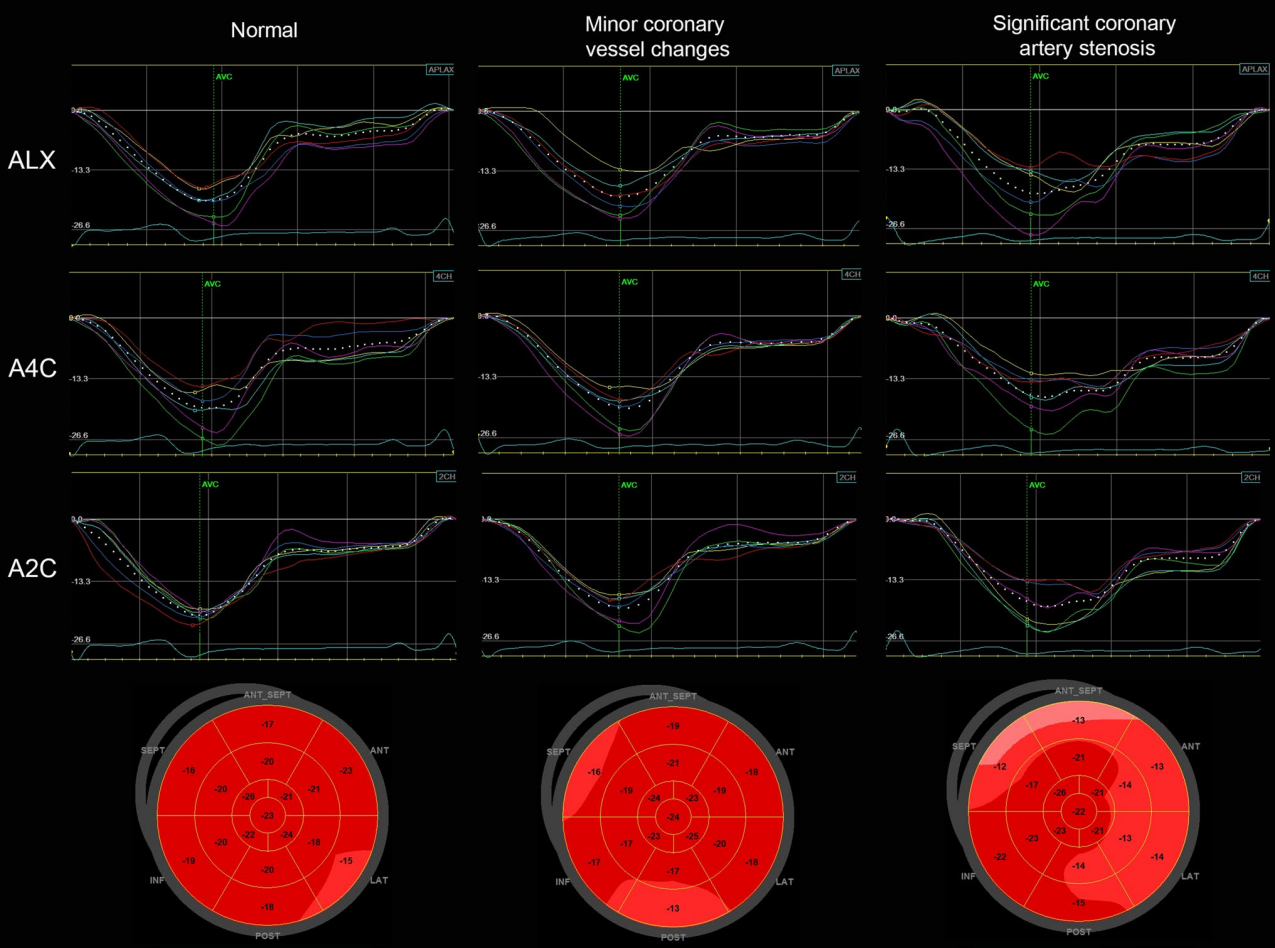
Left column: an example of one patient with normal coronary arteries. GLS: − 20.2%, 4AS: − 17.8%. Middle column: an example of one patient with minor coronary vessel disease—GLS: − 19.7%, 4AS: − 16.0%. Right column: an example of one patient with a significant (70–89%) coronary artery stenosis in the left anterior descending artery. GLS: − 18.5%, 4AS: − 13.5%. *ALX* apical long axis, *A4C* apical four-chamber, *A2C* apical two-chamber, *GLS* global longitudinal strain, *4AS* regional longitudinal strain of four adjacent segments
